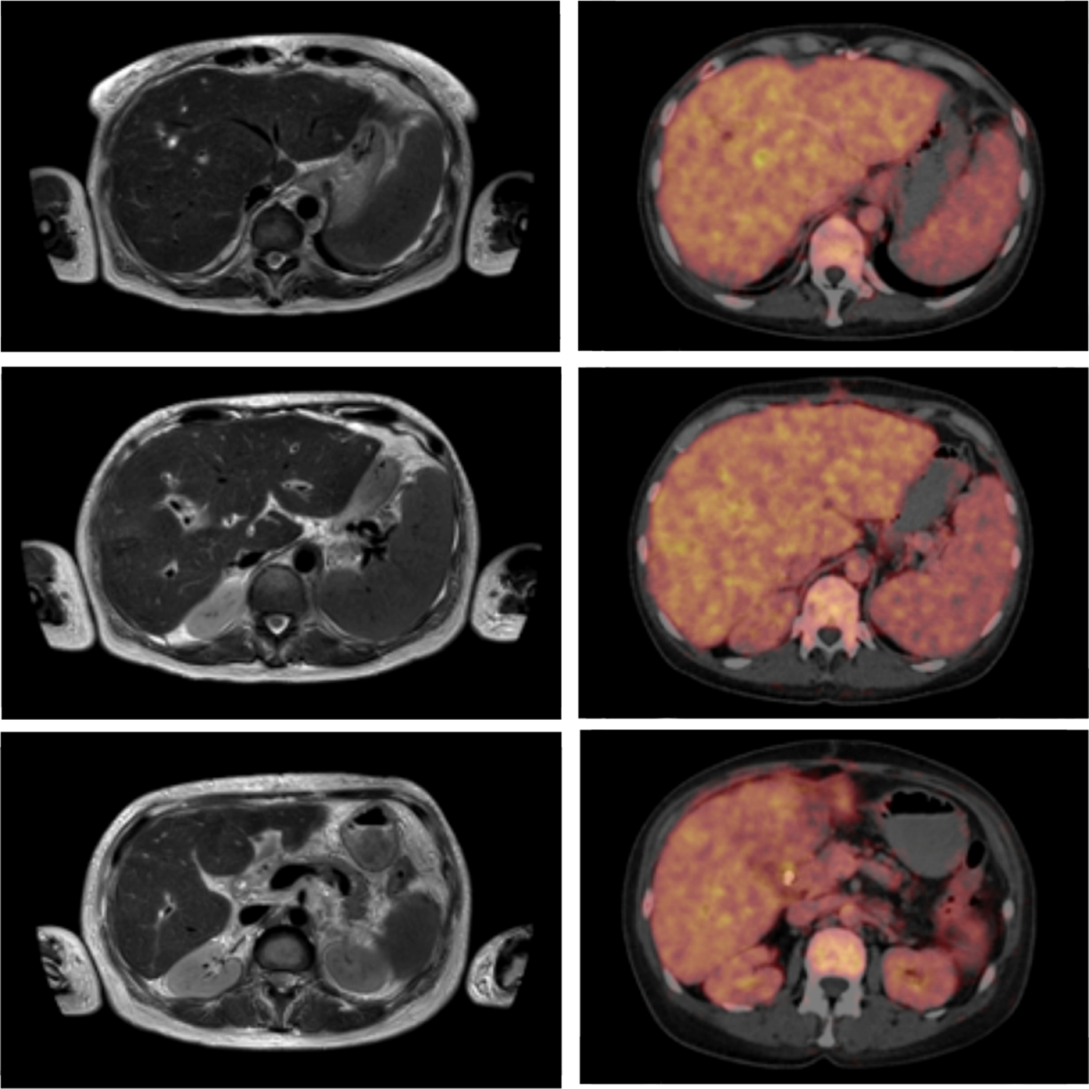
Follow-up imaging by MRI and PET/CT six weeks after discharge
